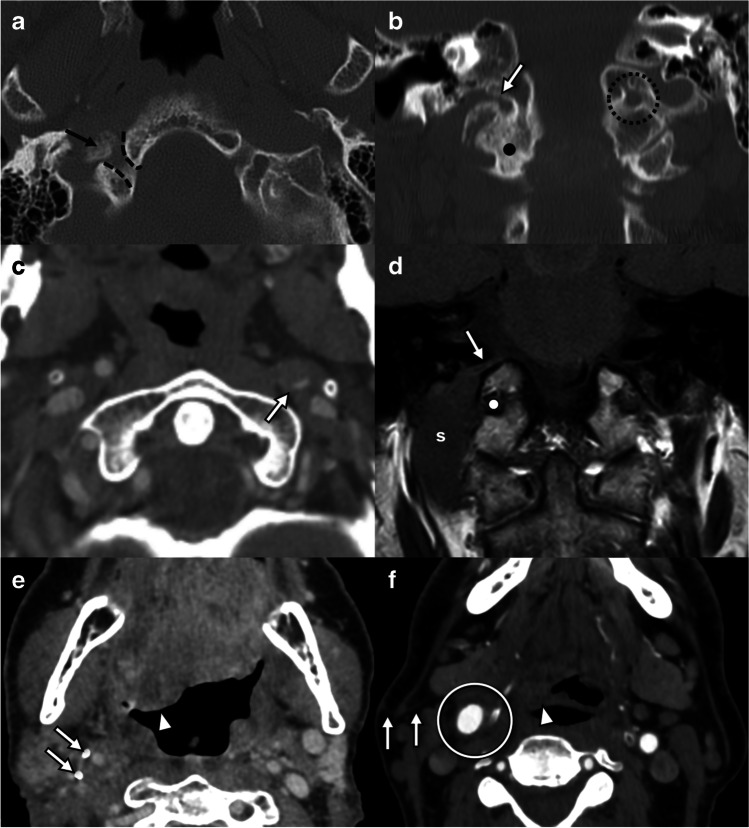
Lesions involving the carotid segment of the hypoglossal nerve. Further tumors of the carotid sheath have been presented in Fig. 1. a Axial unenhanced CT in a 76-year-old female with prior cervical fusion surgery presents with right tongue weakness after trigger point injections reveals an osteophyte (arrow) overlying the external os of the right hypoglossal canal (dashed line). b Coronal unenhanced CT in the same patient as a reveals ankylosis of the right C1-C2 articulation contiguous with the osteophyte (dot) causing stenosis of the right hypoglossal canal (arrow). The left hypoglossal canal (circle) is normal in caliber. c Axial contrast-enhanced CT angiogram in a 44-year-old presenting with neck pain and tongue weakness demonstrates irregular narrowing of the left cervical internal carotid artery lumen consistent with a dissection flap (arrow). Left tongue ptosis was shown in Fig. 1c. d Coronal T1W in the same patient as Fig. 1f, a 54-year-old patient with tongue weakness and episodic dizziness, demonstrates a fusiform T1 hypointense schwannoma (s) arising from pars nervosa of the right jugular foramen (arrow) and causing extrinsic mass effect on the right hypoglossal canal (dot). Further tumors of the carotid sheath have been presented in Fig. 1. e Axial contrast-enhanced CT neck in a 62-year-old 3 months after salvage right neck dissection for nodal recurrence of oropharyngeal SCC demonstrates surgical clips in the right carotid space (arrows) and effaced suprahyoid fat planes due to post-treatment changes as well as acute right hypoglossal denervation (arrowhead). There was no evidence of perineural disease on MRI (not shown). f Axial contrast-enhanced CT angiogram of the neck in a 61-year-old 1 month after right carotid endarterectomy demonstrates linear scarring (arrows) and loss of fat planes (circle) in the right carotid sheath due to post-surgical changes and acute right hypoglossal denervation (arrowhead). Follow-up CT angiogram for planning of contralateral endarterectomy demonstrated resolution of the right hypoglossal palsy (not shown)
